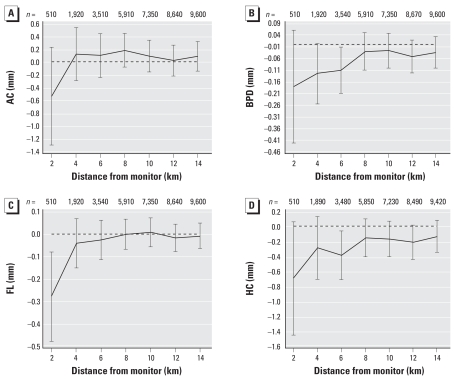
Change in ultrasound measurements (mm) for a 5-μg/m^3^ increase in PM_10_ during days 0–30 of gestation by distance to monitor. (*A*) AC; (*B*) BPD; (*C*) FL; (*D*) HC.
